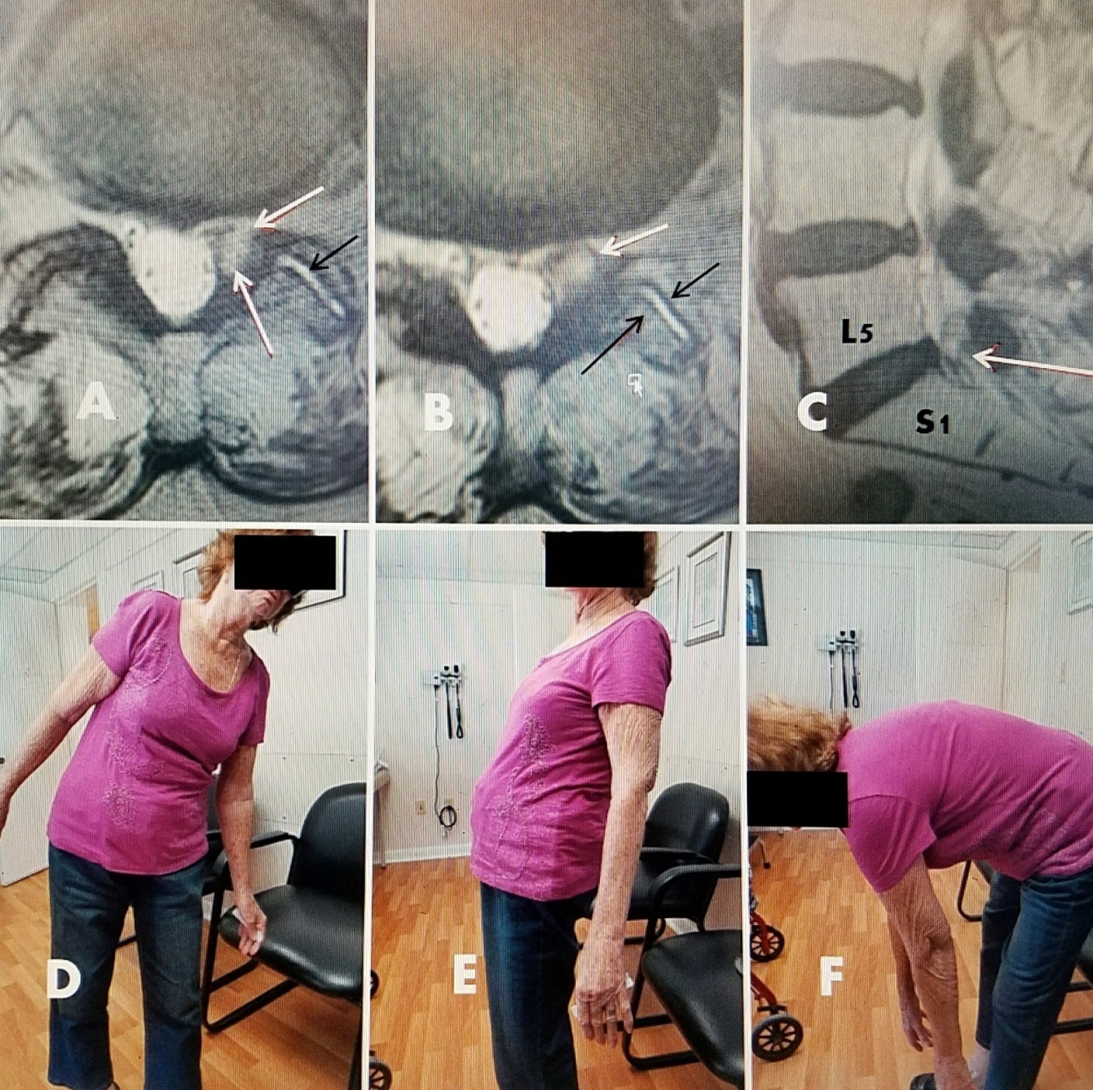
79-year-old female with L5-S1 foraminal cyst with radiculopathy A, B: T2 axial MRI showing left foraminal facet cyst (large solid white arrow) and hyperintense fluid in left facet joint (small black arrow). C: Sagittal T2 MRI showing posterolateral cyst at L5-S1 (white arrow). D: Patient 18 months after drainage and ablation with lateral bending to the left, towards side of cyst. E/F: 18 months after RF cyst ablation. Patient has recovered full movement with extension and flexion without limitation or leg pain.
